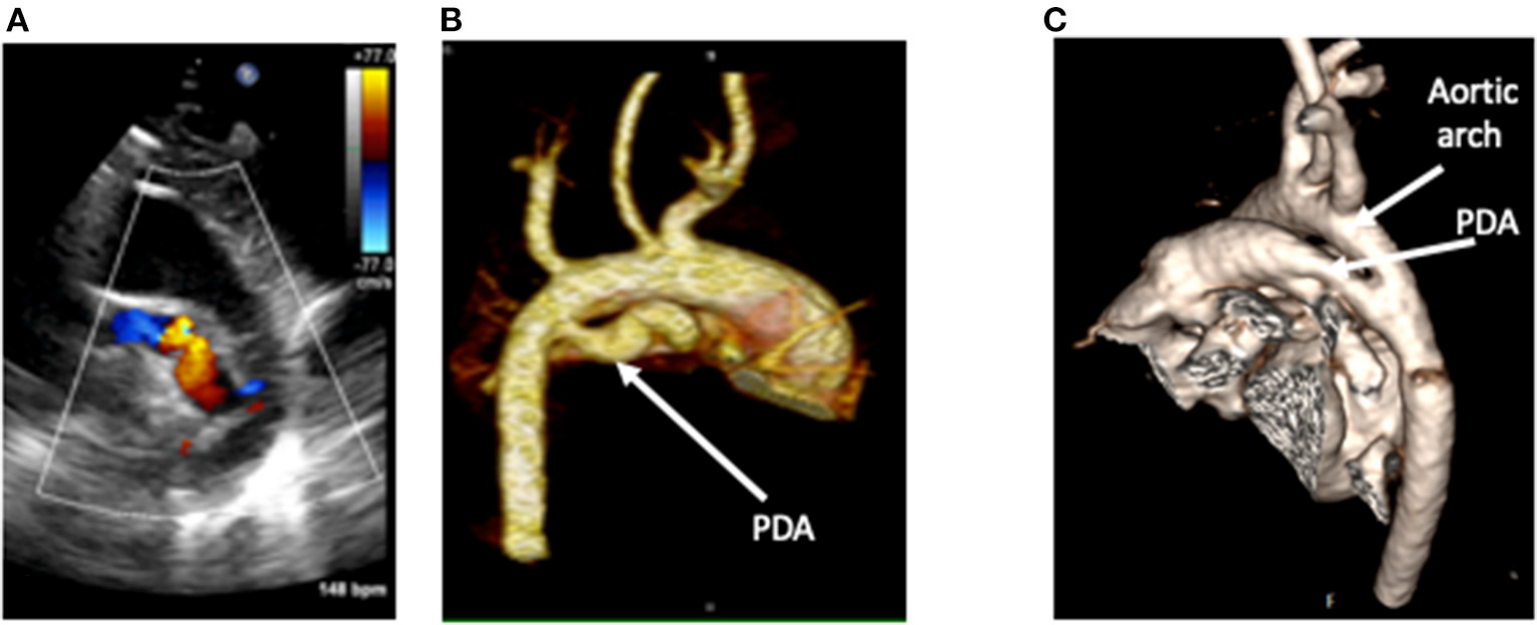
A tortuous duct as seen on 2D color Doppler echocardiography (A), with clear delineation of the shape, dimensions, and insertion points on contrast-enhanced CT (B). This is in comparison to a straight duct seen on CT in image (C). CT Images courtesy of Dr. Sujeev Mathur, Evelina London Children's Hospital.
